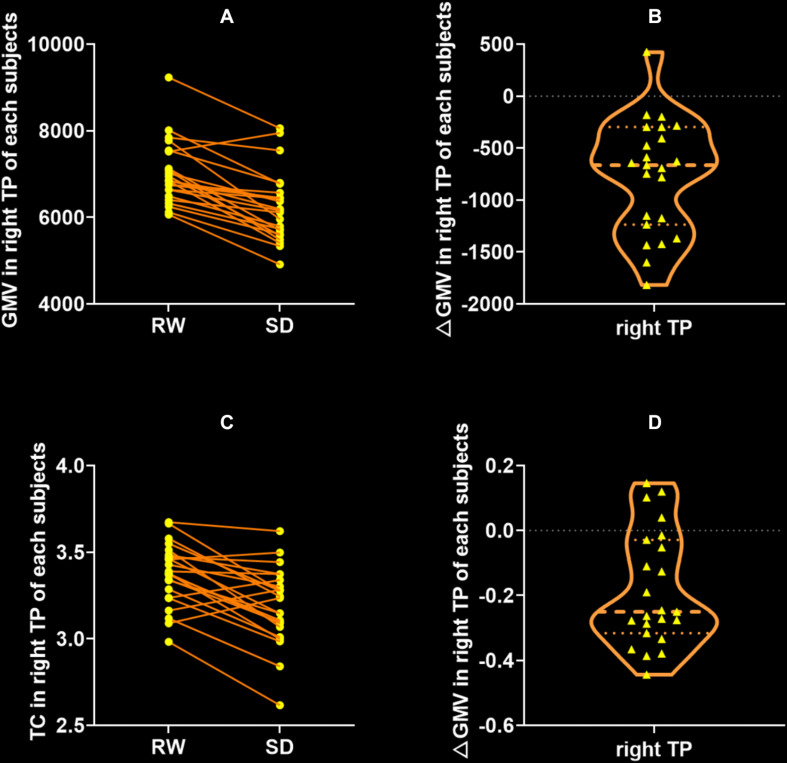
Significant decrease of gray matter volume (GMV) and cortical thickness (CT) between after SD state. The only significant brain region of GMV (A,B) and CT (C,D) was the right temporal pole (TP). There were no regions that exhibited significant change of GMV or CT after SD. RW, rested wakefulness; SD, sleep deprivation.
